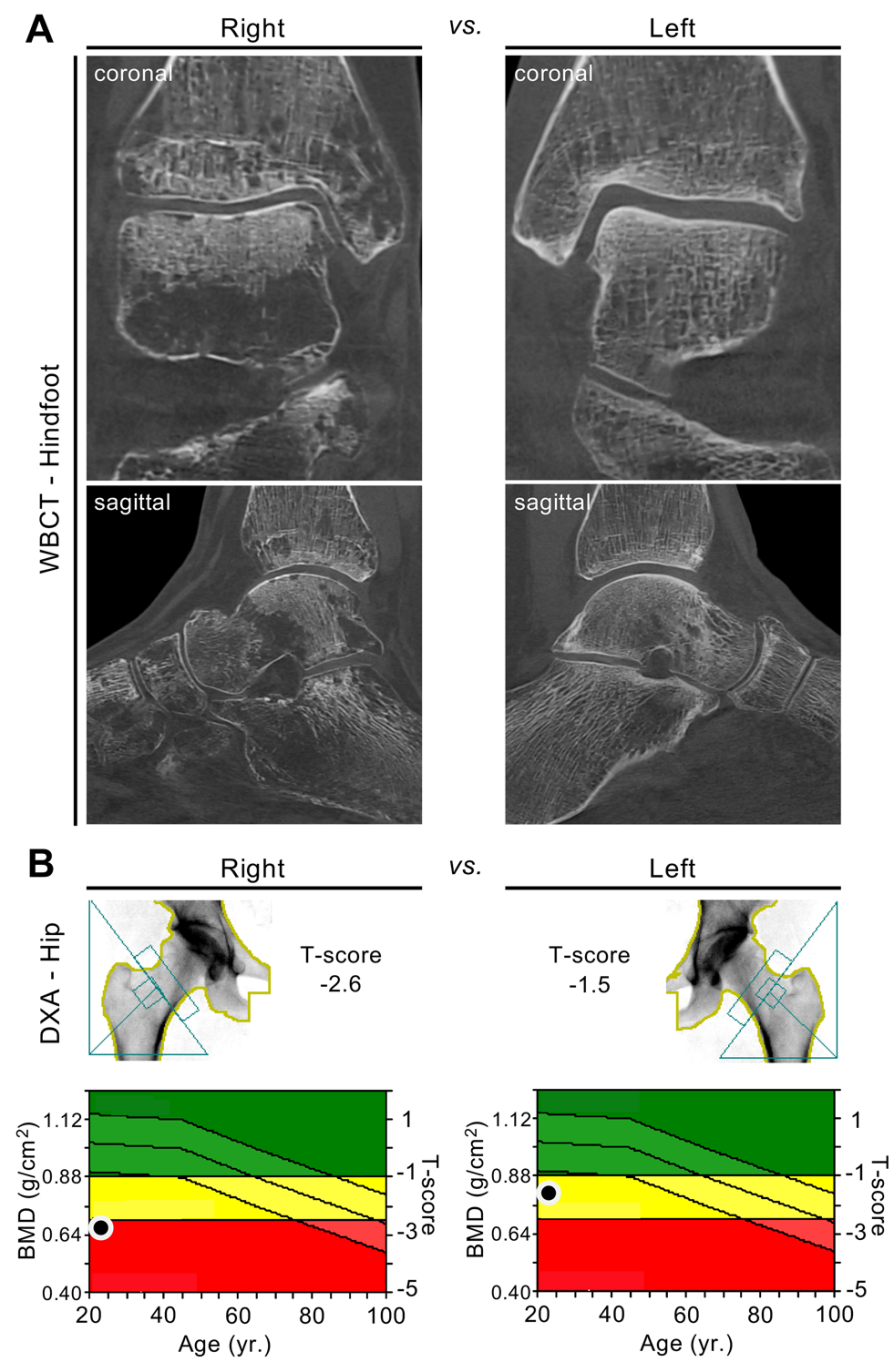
Local deterioration of bone mass after fracture and subsequent unilateral disuse of the right limb. a Cone-beam computed tomography (CBCT) images of the right foot compared to the left foot (coronal and sagittal reconstruction) in a 22-year-old female patient obtained eight months after suffering from an ankle fracture with subsequent unilateral unloading of the right limb. Focal osteolytic changes (often referred to as “local disuse osteopenia/osteoporosis”) are visible. b DXA scans demonstrating the differences in BMD between the right and left proximal femur (T-score − 2.6 vs. − 1.5). Bilateral HR-pQCT scans of the distal tibiae were also performed and showed cortical and trabecular bone loss syndrome on the unloaded side compared to the contralateral (loaded) side (cortical thickness − 28%, bone volume per tissue volume − 7%)
